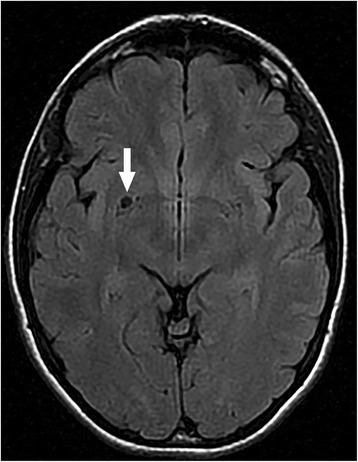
Magnetic resonance imaging (MRI) scan. Dilated atypical Virchow-Robin space (VRS) under the basal ganglia on the right side is visible (white arrow)
